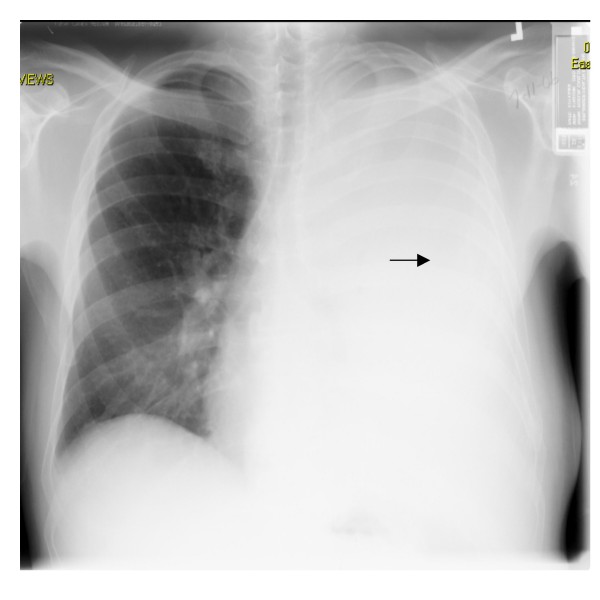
Chest X-ray (PA view): Re-accumulation of massive left sided pleural effusion (arrow).
